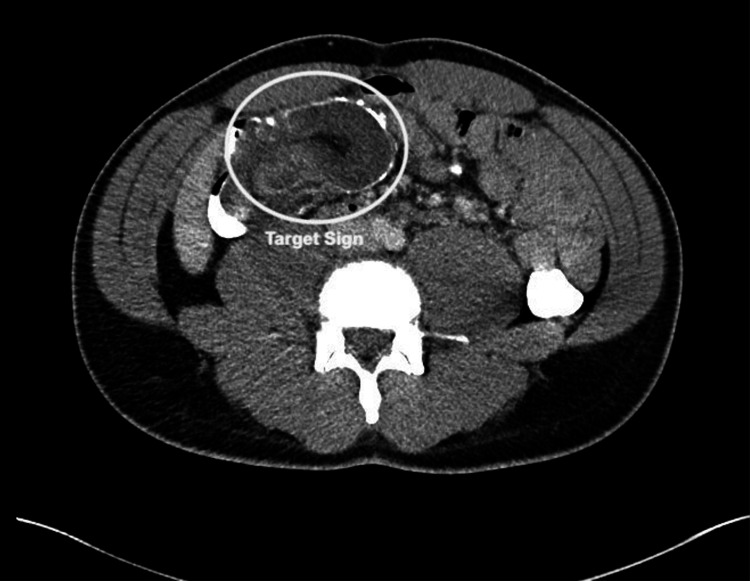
Axial CT scan of the abdomen with IV contrast showing the classic "target sign" of ileocolic intussusception.
